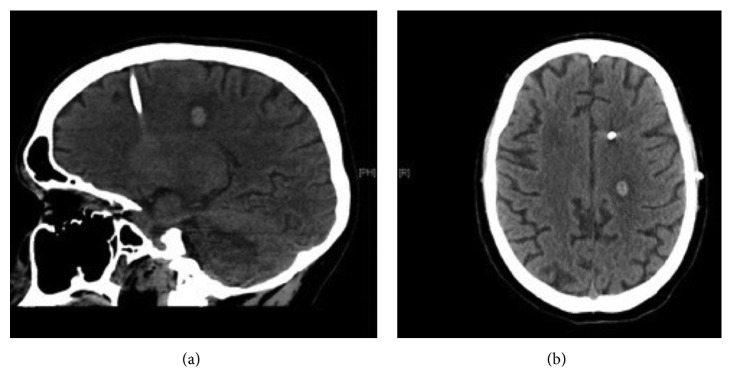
CT scan showing the new cerebral focal lesion.
